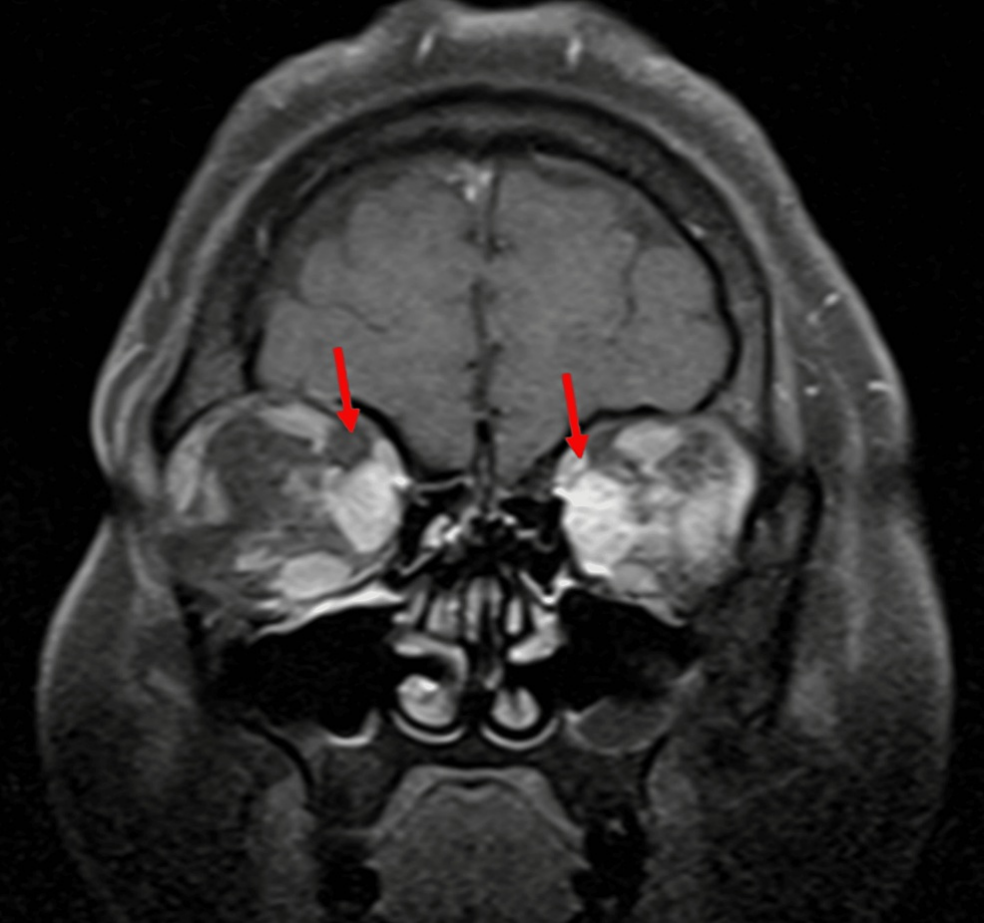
Orbital MRI (T1, coronal view) showing significant enlargement of extraocular muscles (bilateral medial recti indicated by red arrows) MRI: magnetic resonance imaging
